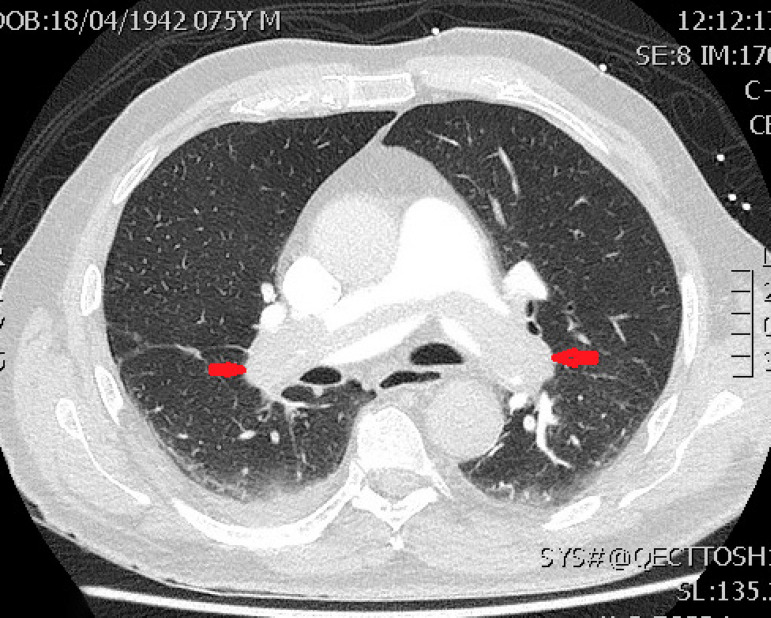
Computed tomography of pulmonary artery (axial view). Arrows show saddle
